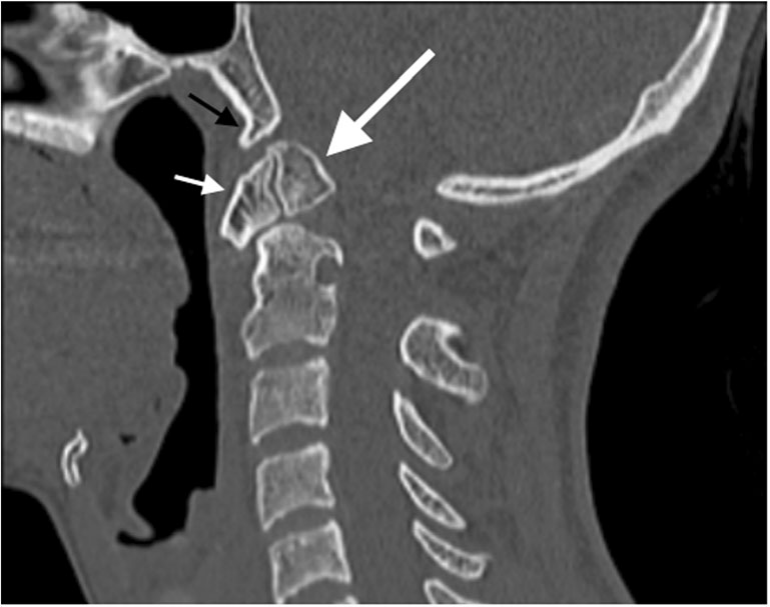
Os odontoideum: sagittal reformatted CT image of the craniocervical junction performed as part of a trauma CT assessment of a young female victim of a fall from height demonstrates a pre-existing os odontoideum (*large white arrow*) mimicking a type II odontoid process fracture. While the (type II) odontoid fracture is typically associated with a flattened uncorticated sharp margin to the adjacent body of the axis and a normal morphology to the anterior arch of the atlas, the os odontoideum exhibits a well-corticated convex upper margin. A commonly associated rounded hypertrophic anterior atlas arch is also present (*small white arrow*). This, together with an associated condylus tertius (*small black arrow*) which represents a remnant hypochordal bow, further confirms the non-acute traumatic aetiology of the odontoid process appearances
